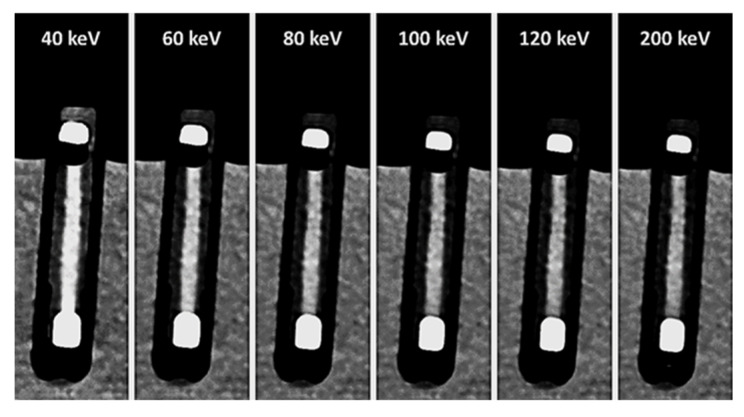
Depiction of the same thrombus (40% RBC content) in virtual monoenergetic images at six different energy levels: 40 keV, 60 keV, 80 keV, 100 keV, 120 keV and 200 keV. Particularly between 40 keV and 80 keV, a visual decrease in CT density is apparent. With higher energy levels, a further visual decrease in CT-density applies only to a very slight extent at best.
